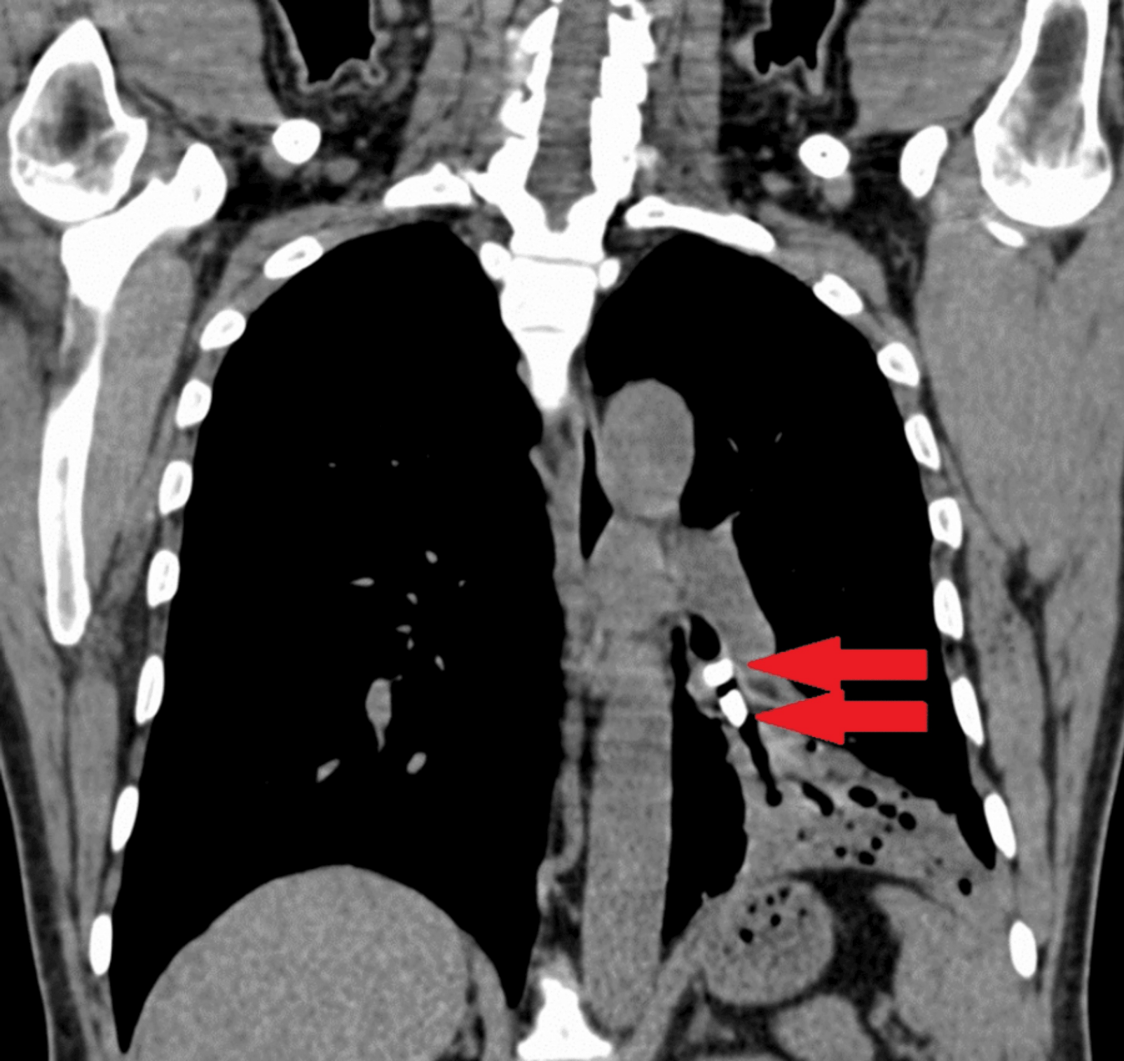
High-resolution computed tomography of the chest mediastinal window coronal section revealed two well-defined bone density areas (HU+1850 to +1980) seen within the left lower lobe bronchus, one measuring 11 x 8 mm and the other measuring 8 x 6 mm (red arrows).
